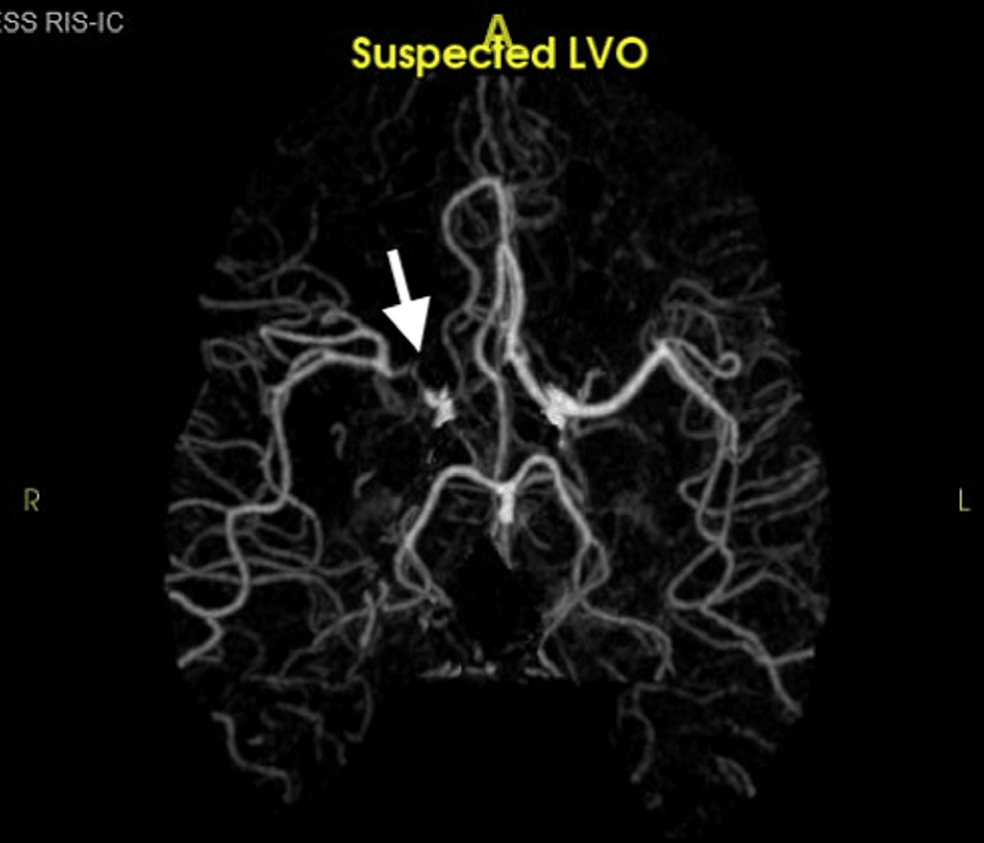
CT angiogram of the brain. A right mid-posterior division M2 middle cerebral artery vessel branch demonstrates high-grade long-segment stenosis. LVO: large vessel occlusion.
